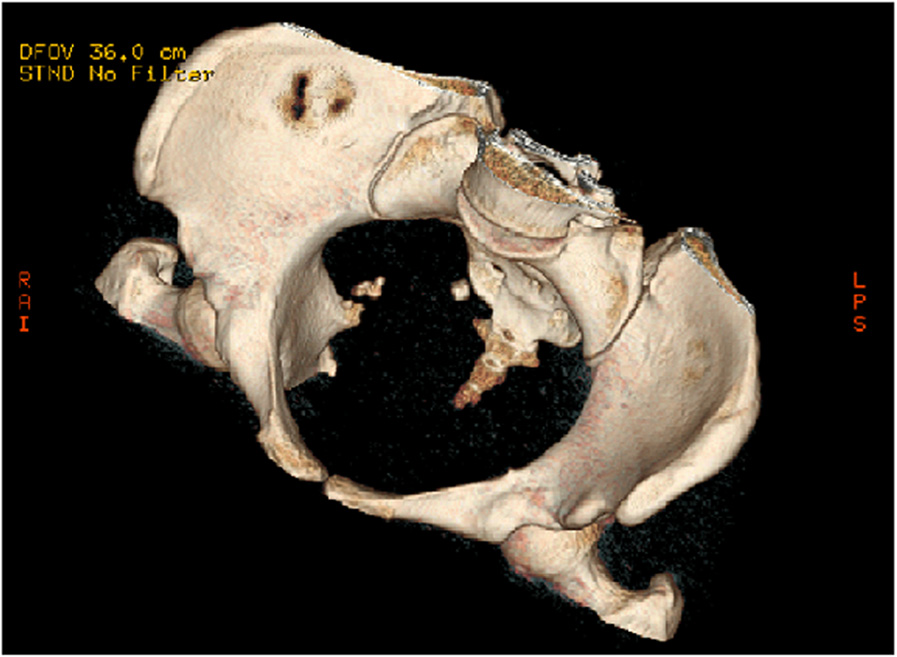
Two separate bony nodules were showed in the pelvic cavity by three-dimensional multislice computed tomography (MSCT-3D) examination.
